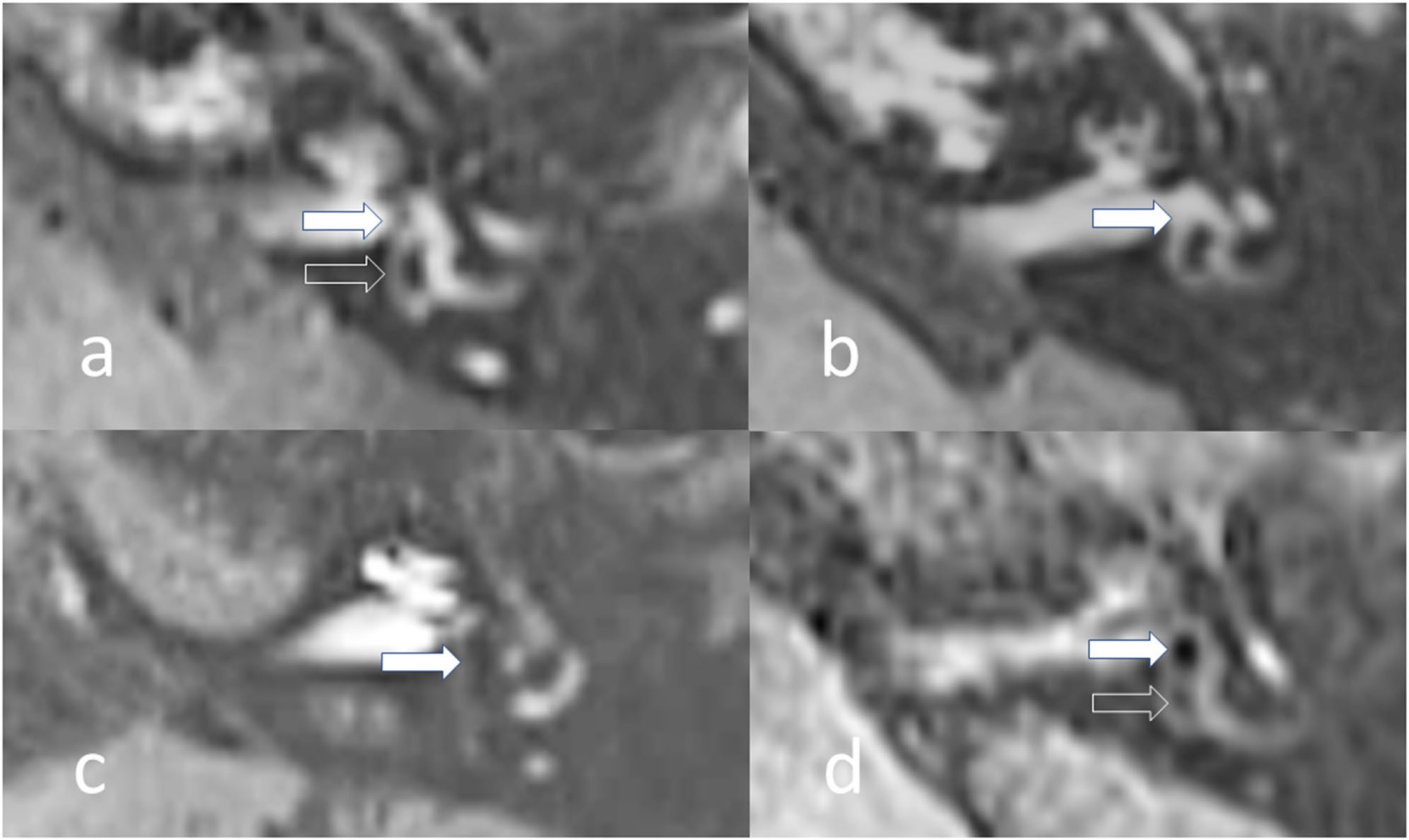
Delayed post-gadolinium 3D-real IR axial images angled through the inferior vestibule demonstrating saccule-related MRI descriptors. a Normal appearances with the smaller saccule (filled arrow) anterior to the utricle (open arrow). b Absent saccule with the expected location indicated (filled arrow). c Saccule confluent with the utricle (filled arrow). d Saccule (filled arrow) larger than the utricle (open arrow)
